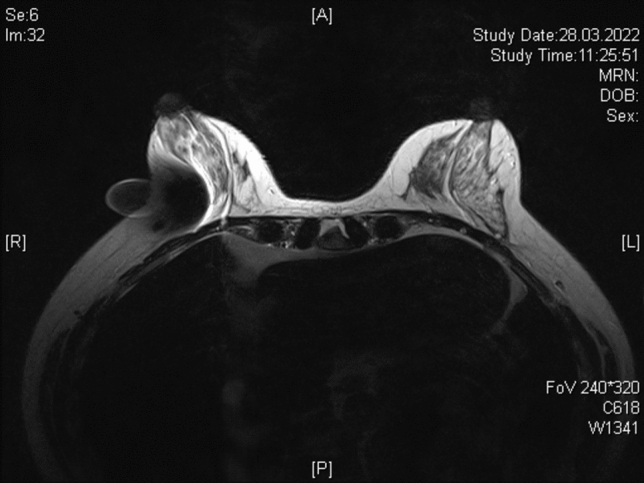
Impairment of breast MRI assessment due to an artifact caused by an axillary magnetic seed in craniolaterally located cancer of the right breast
